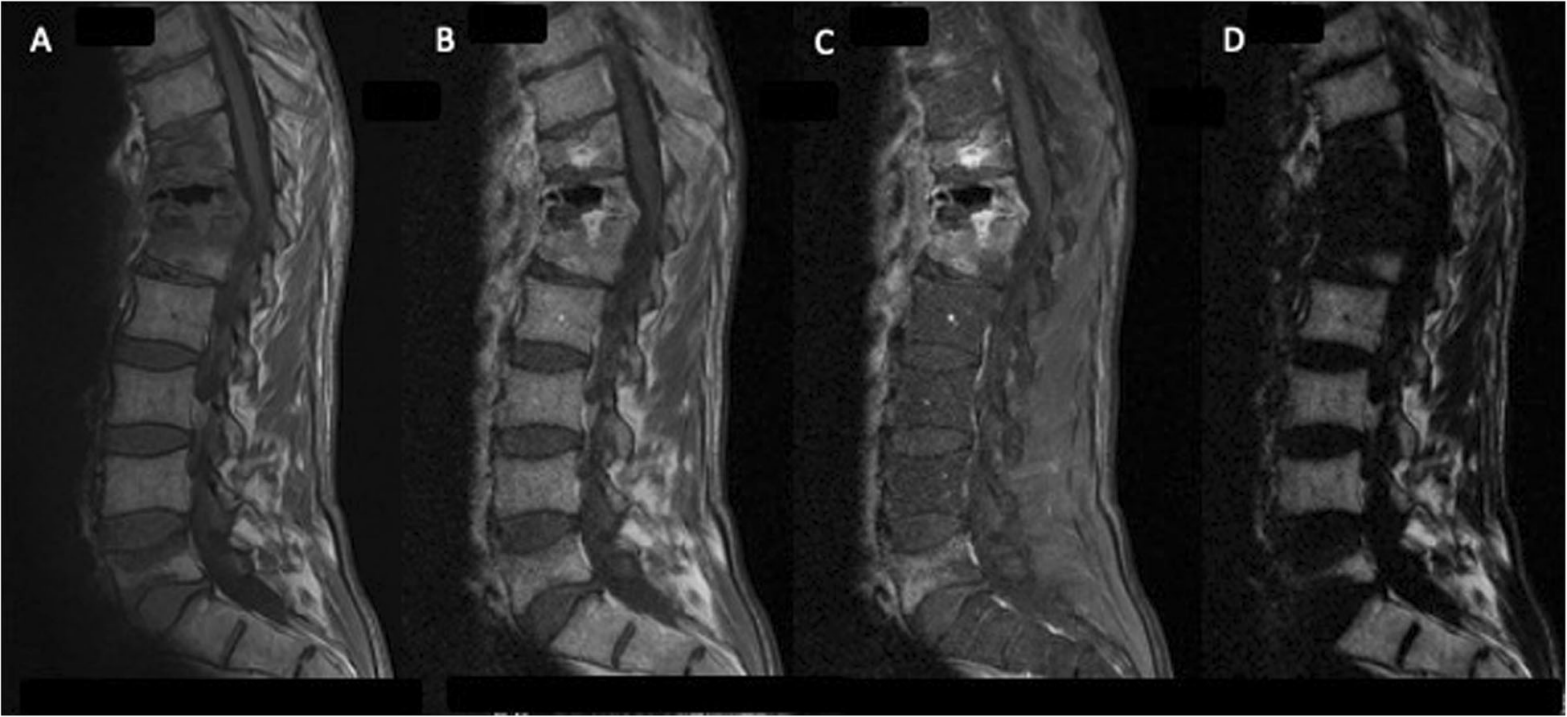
Spinal MRI, sagittal section: Hyperintensity, T11-T12-L1 vertebrae, para-vertebral soft tissue, and T12-L1 disc consistent with infectious spondylodiscitis. a T1-weighted; b, c, d IDEAL sequence
